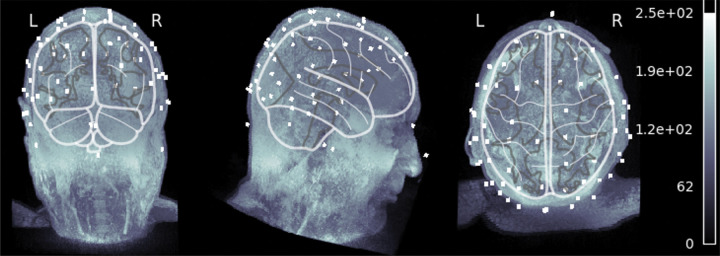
Three-Dimensional EEG Electrode Localization and Cortical Mapping Over MRI Brain Reconstruction [14,15]
